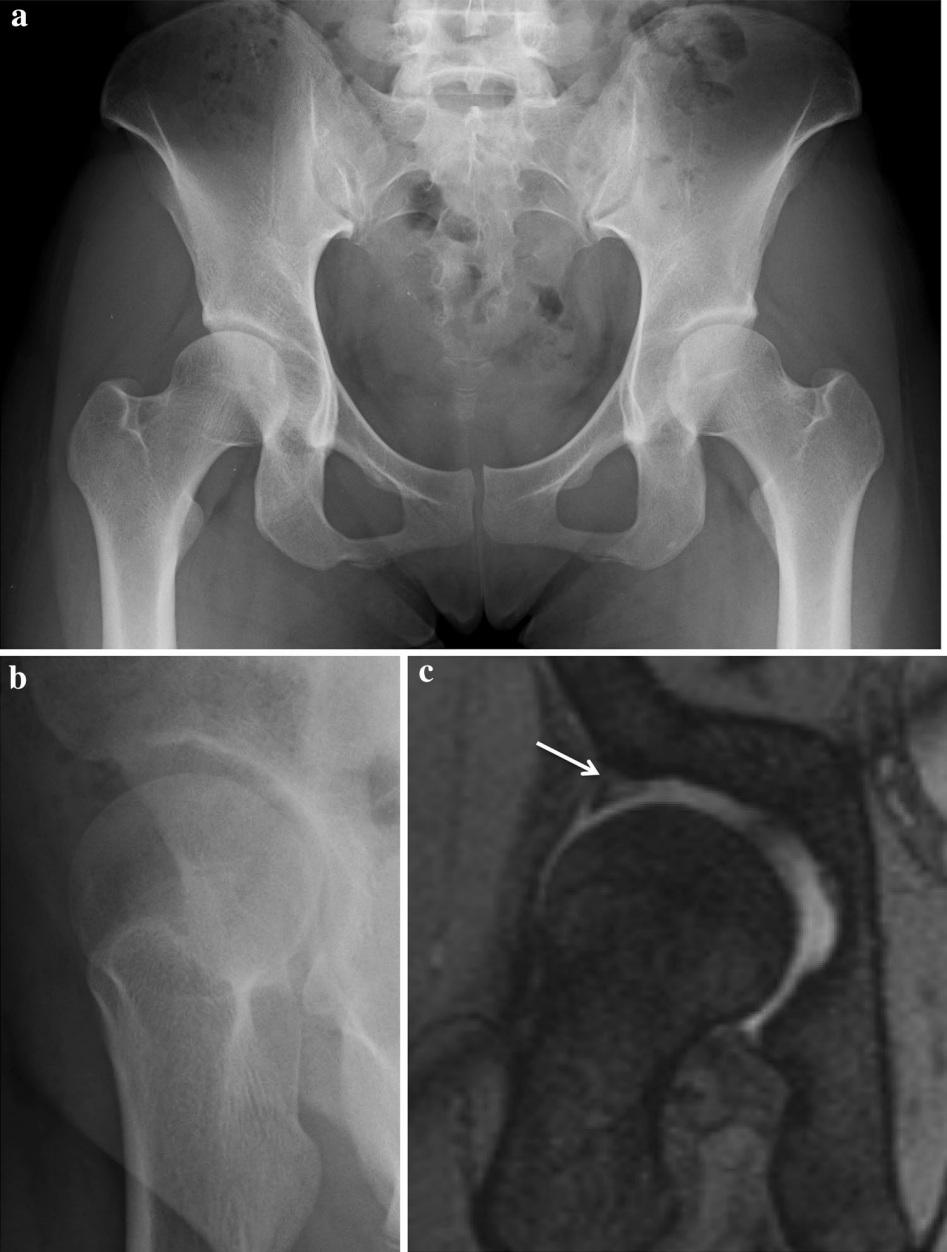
A 17-year-old speed skater presented to us with a 6 month history of right hip pain. a Diagnostic preoperative pelvic AP radiograph shows DDH. The center edge angle is 16° and the sharp angle is 49°. b Preoperative false profile view also shows anterior shallowness of acetabulum. The vertical–center–anterior (VCA) angle is 18°. c T2-weighted magnetic resonance imaging showing an area of high at acetabular labrum, suggestive of a possible acetabular labral tear
